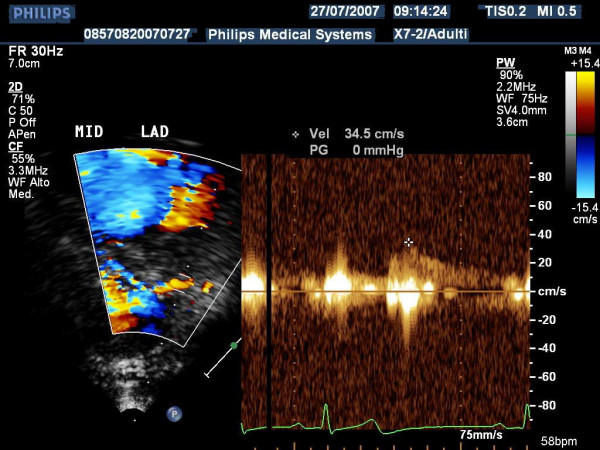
shows the Pulse Doppler envelope of mid tract of left descending coronary artery.
